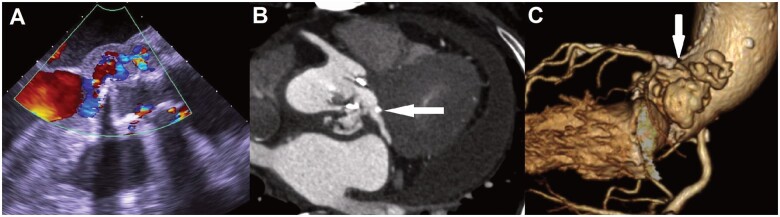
(A) Preoperative transoesophageal echocardiography showed the abscess with blood flow in the intervalvular fibrosa. (B, C) Preoperative computed tomography demonstrated the ruptured left ventricular outflow tract with contrast extravasation behind the aortic root and ascending aorta (arrow).
